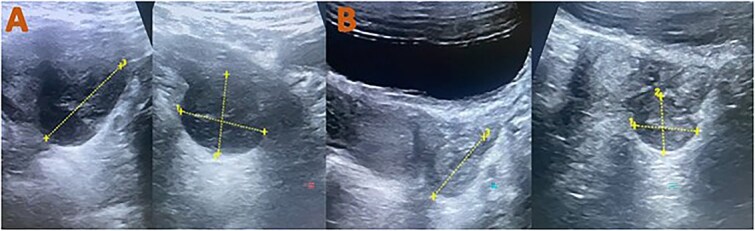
Follow-up pelvis ultrasound exams: (A) after 2 weeks the previously visualized left adnexal mass became more circumscribed and showed internal cystic texture measured (30 × 28 × 37 mm = 16 cc volume). (B) 6 weeks later: The mass had decreased in size and developed a heterogeneous echogenic texture, measuring 13 × 16 × 24 mm (3 cc volume).
